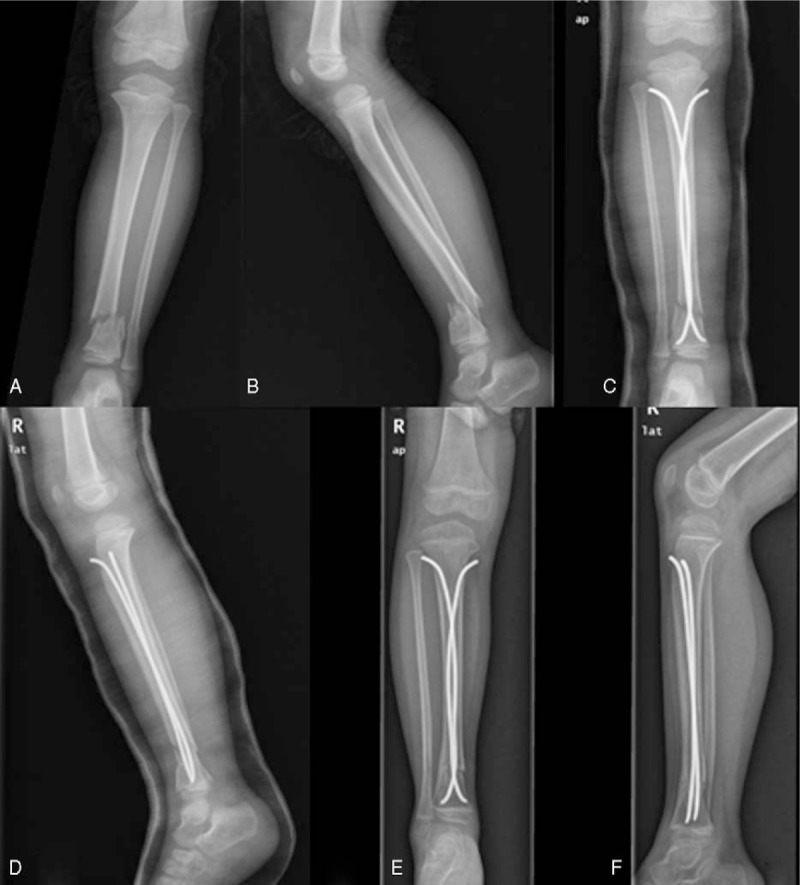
A 6.1-year-old girl fell down from 1.5 m height. A, B, Anteroposterior and lateral views of the severe right DTDMJ fracture, mild oblique type. C, D, Immediate postoperative radiographs after closed reduction and fixation with 2 nails. E, F, 3 months after surgery, the fracture showed radiographic evidence of healing. DTDMJ = distal tibial diaphyseal metaphyseal junction.
